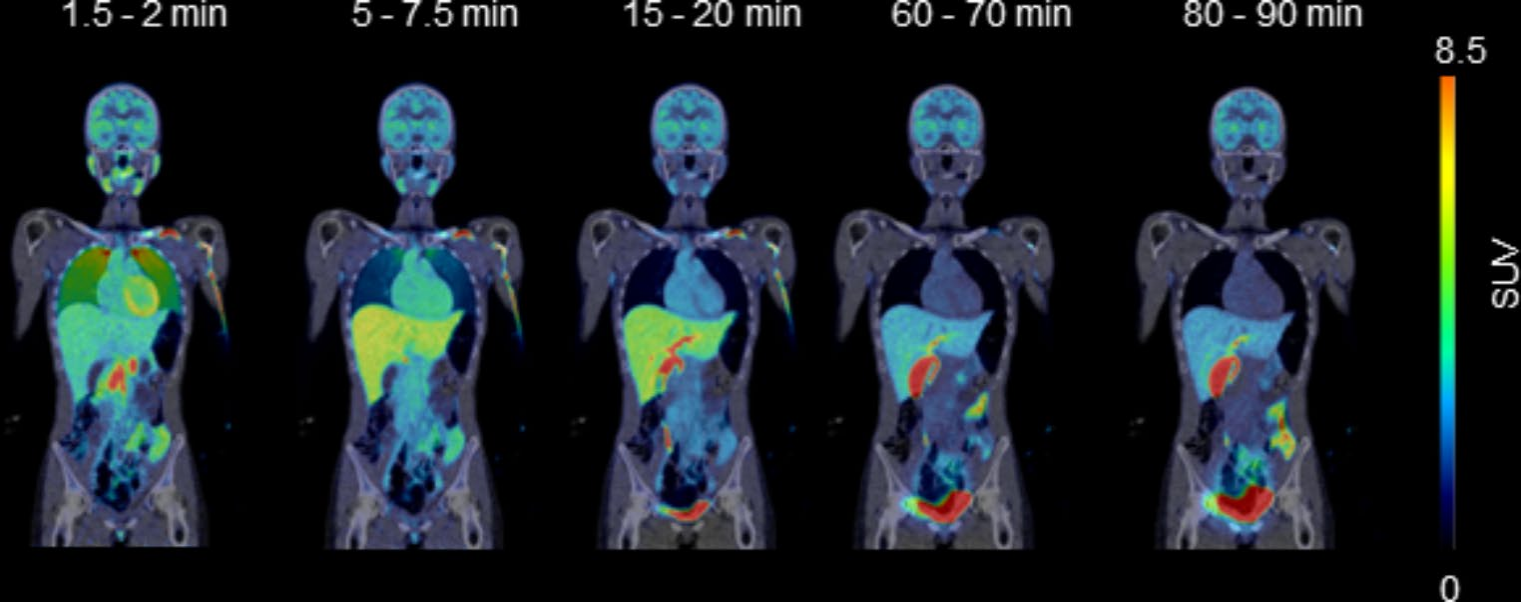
Coronal whole-body PET/CT images obtained on the LAFOV scanner (axial FOV: 106 cm) at different time points after injection of 389 MBq [^11^C]BMP in one representative female subject (25 years, 66 kg). Activity scale is given in SUV units and set from 0 to 8.5
